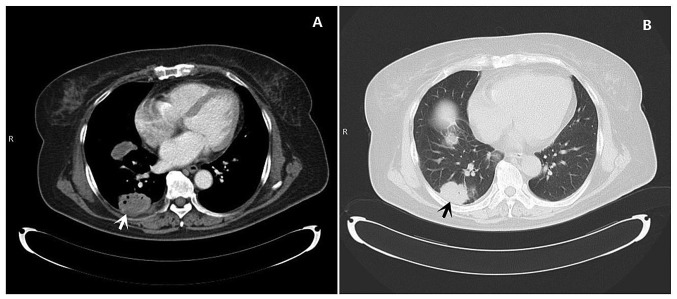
Chest computed tomography showing the primary tumor in the inferior lobe of the right lung (arrow) in the (A) mediastinal and (B) pulmonary windows.
